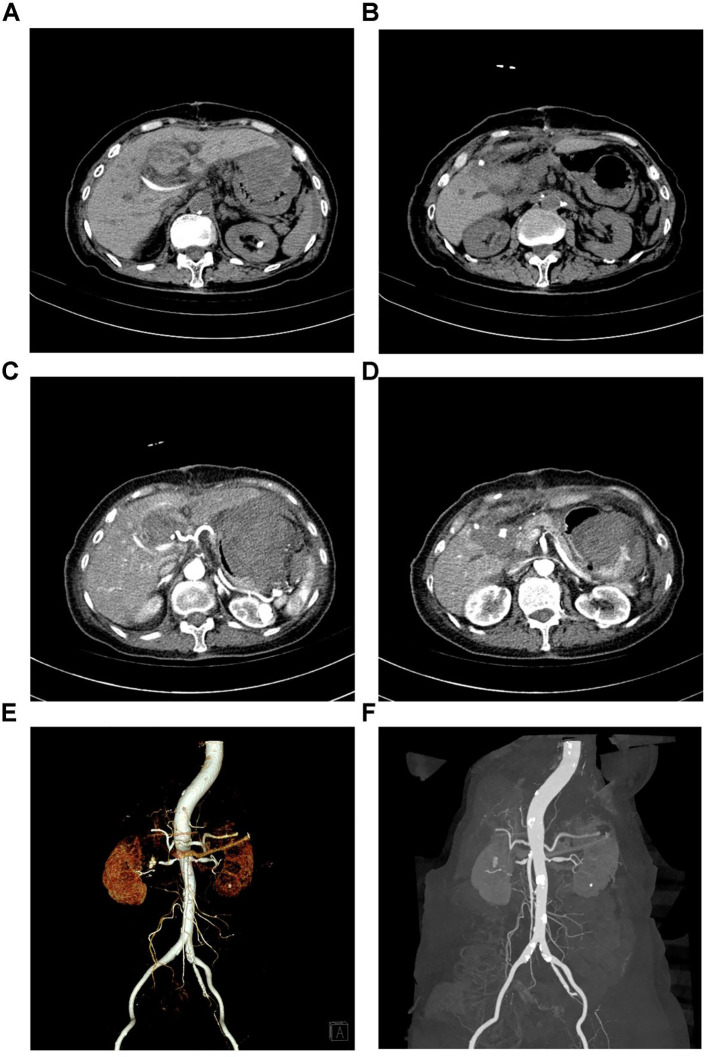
Imaging examinations during the patient’s intra-abdominal hemorrhage. (A) Plain CT scan revealing a hematoma between the lesser curvature of the stomach and the left liver. (B) Plain CT scan showing a hematoma at the porta hepatis. (C) Abdominal arterial CTA showing that the hematoma on the lesser curvature side and at the porta hepatis was significantly larger than revealed by plain CT. (D) Abdominal arterial CTA image indicating the formation of a pseudoaneurysm within the hematoma at the porta hepatis. (E, F) Abdominal arterial CTA vascular reconstruction revealed a pseudoaneurysm in the upper right abdomen with contrast agent leakage outside the vessels.
